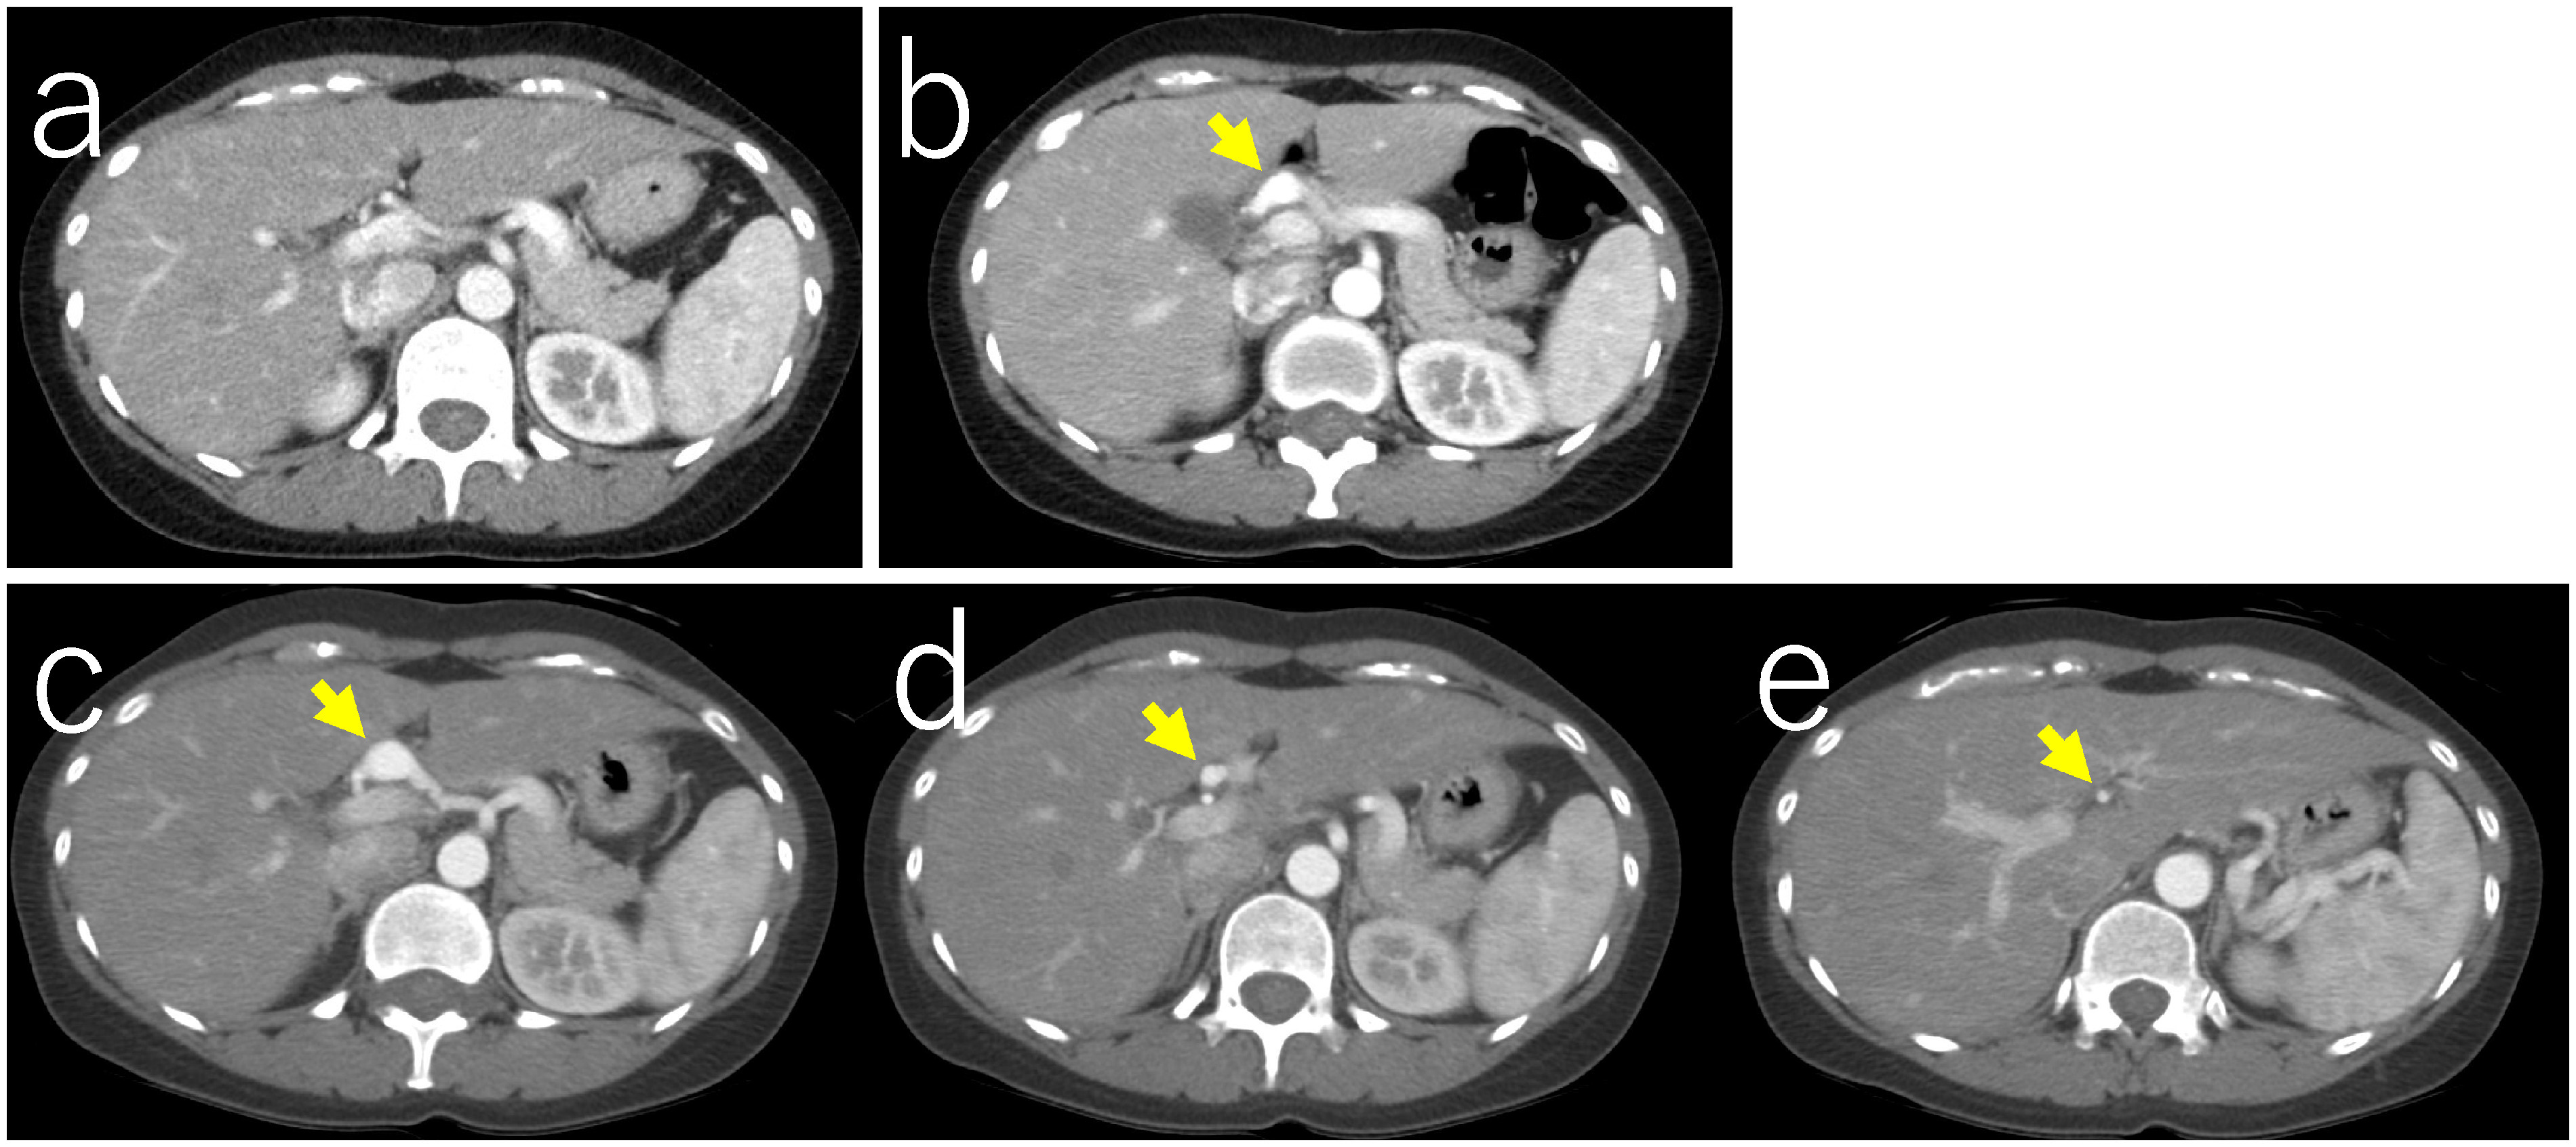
Fig. 1 Contrast-enhanced computed tomography (CT) images showed the rapid expansion of arterial aneurysms. (a) At a regular consultation 11 days before the patient’s admission, regular CT once a year at our hospital showed no aneurysm. (b) CT image on admission demonstrating a common hepatic arterial aneurysm (7 mm in diameter) (arrow). (c–e) Follow-up CT image on day 7 of the patient’s hospitalization showing an expansion of a common hepatic arterial aneurysm (14 mm in diameter), a new proper hepatic arterial aneurysm, and new left hepatic arterial aneurysms (arrow).
